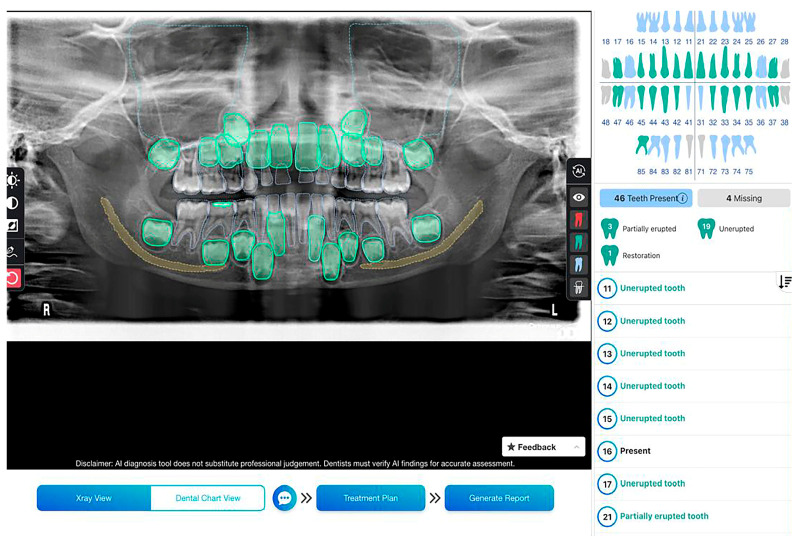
Panoramic radiograph of a 6-year-old subject showing mixed dentition analyzed using EM2AI for primary teeth detection.
